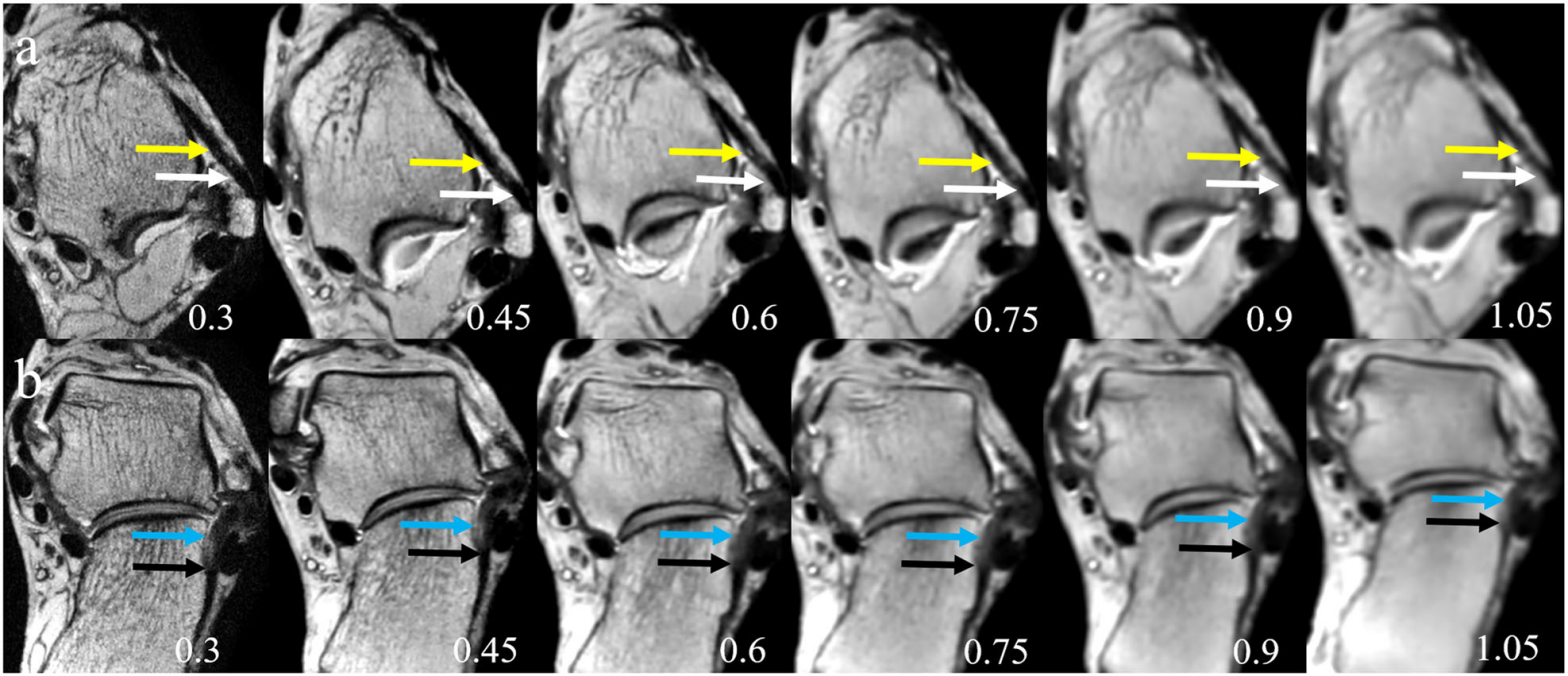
Three-dimensional magnetic resonance imaging reconstruction, showing the entire length of anterior talofibular ligament (ATFL) (a, axial plane, yellow arrow), calcaneofibular ligament (CFL) (b, oblique coronal plane, blue arrow), the proximal site of ATFL (a, white arrow), and the distal site of CFL (b, black arrow) in all six spatial resolutions. In the spatial resolution of 0.3 × 0.3 × 0.3 mm^3^ and 0.45 × 0.45 × 0.45 mm^3^, the boundary between the ligament and the adjacent cortical bone in the proximal or distal site was clear. In the spatial resolution of 0.6 × 0.6 × 0.6 mm^3^ and 0.75 × 0.75 × 0.75 mm^3^, the boundary between the ligament and cortical bone becomes increasingly blurred. In spatial resolutions of 0.9 × 0.9 × 0.9 mm^3^ and 1.05 × 1.05 × 1.05 mm^3^, the boundary between the ligament and cortical bone was completely indistinguishable. At lower resolution, it was difficult to measure the entire length of ATFL and CFL (from the fibular origin to talar insertion of ATFL, fibular origin to calcaneal insertion of the CFL) and the entire thickness (from medial margin to lateral margin of the ATFL and CFL)
